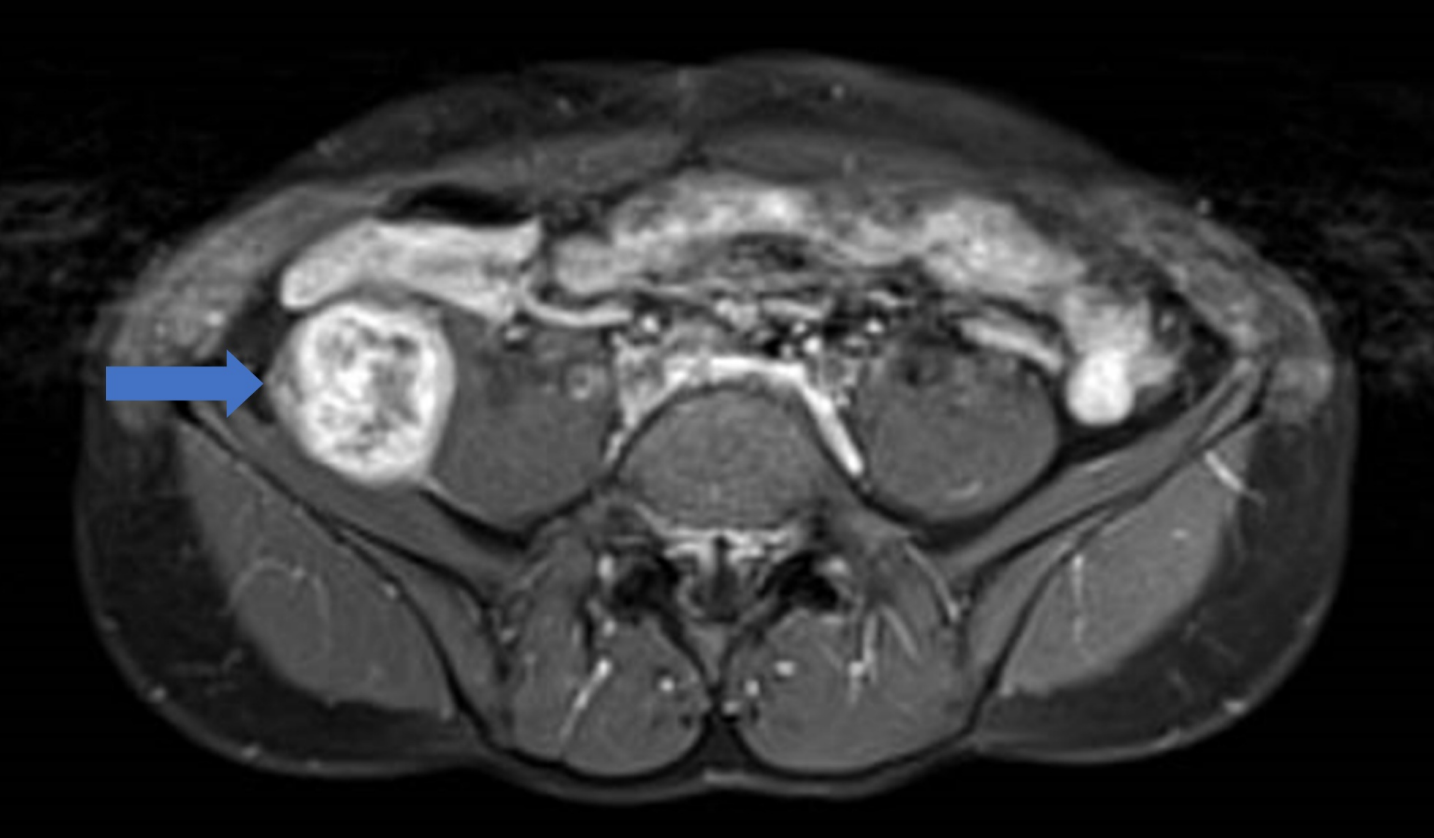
Magnetic resonance image (MRI) of the abdomen with contrast agent. The image is a T1-weighted fat saturation sequence with contrast. It shows the same well-defined lesion in the right iliac fossa with bright heterogeneous enhancement, as well as areas of calcification (blue arrow). The nerve leading to the lesion is much better depicted on MRI. The lesion follows the route of the right L3 nerve root with its distal tail appearing to be communicating with the lateral femoral cutaneous nerve. There was no adjacent fat stranding or edema; the overall appearance would favor a schwannoma over a fibrosarcoma.
